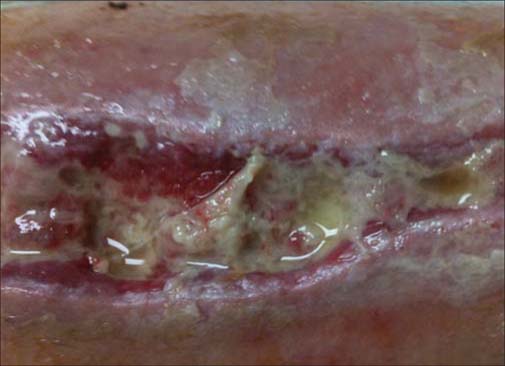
A dehisced surgical incision in a relatively ischemic patient. The opaque film on the wound bed (centre) re-formed daily and could be lifted off to reveal intact granular buds. Ultrasonic debridement was ineffective at disrupting or removing this thick, mature biofilm. Slough is also evident on the intact skin around the wound (top centre, top right, bottom right).
